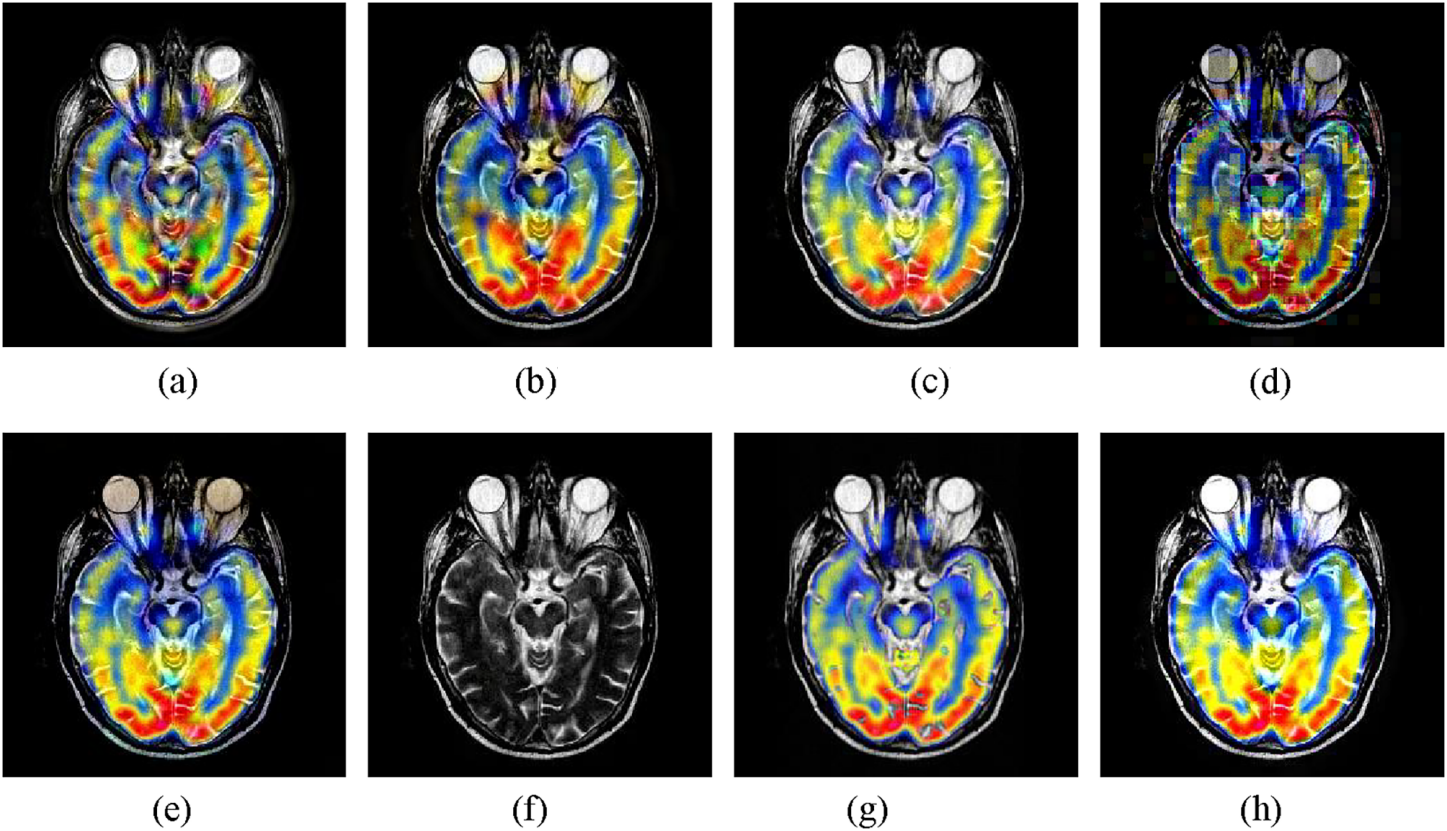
Fusion results of different methods for MRI and PET images. (a) CNN-NSCT. (b) NSST. (c) NSST-ST. (d) SR. (e) MST-SR. (f) GFF. (g) DSWT-RBFN. (h) Our method.
